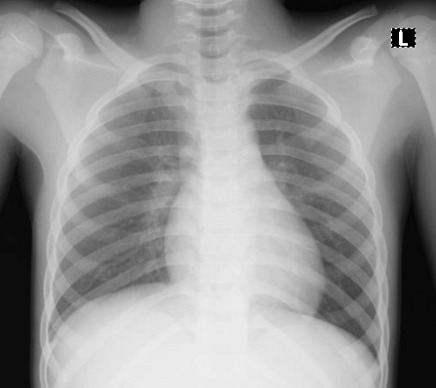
Patient 3 chest radiography showing normal study
